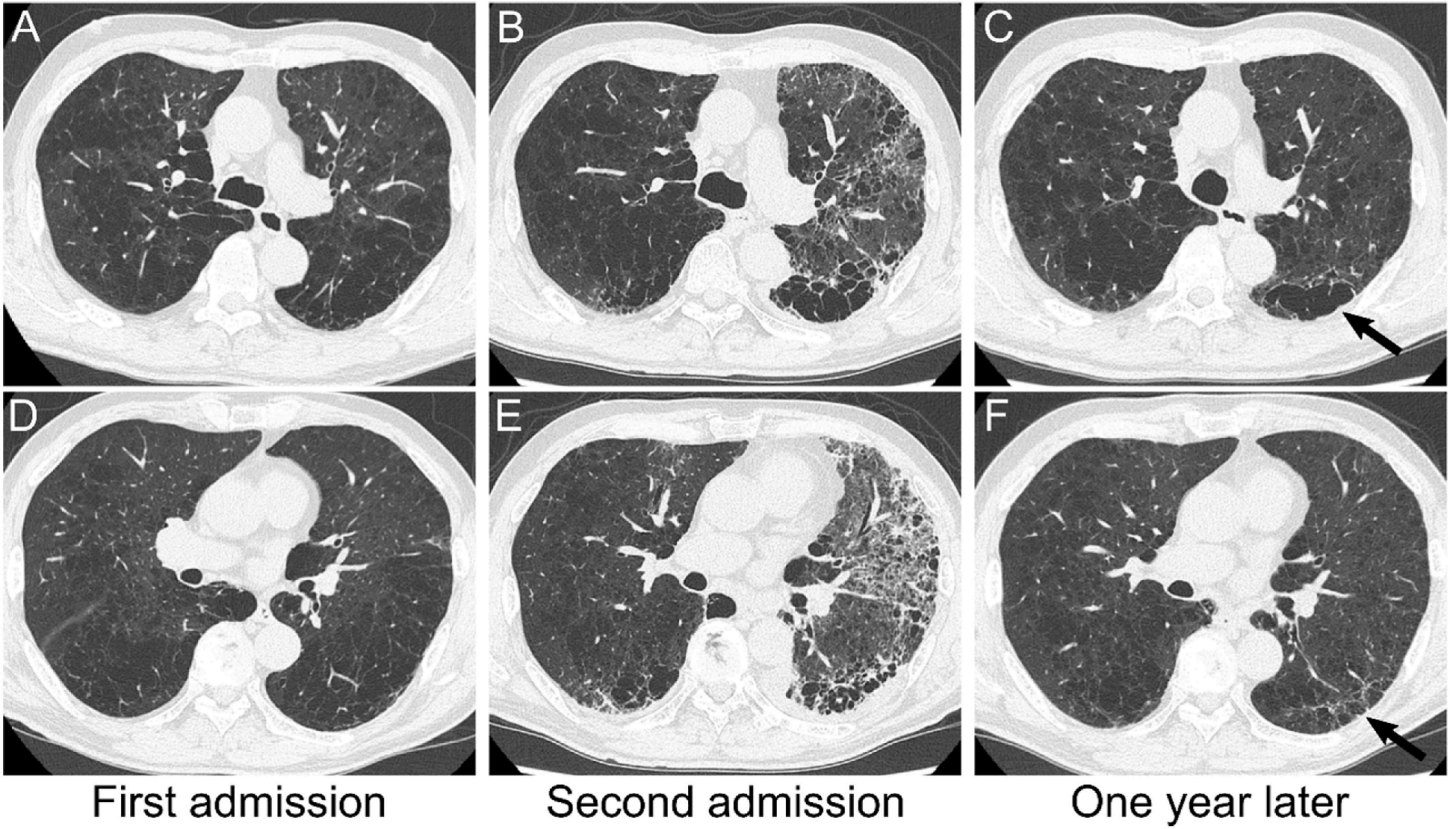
Chest CT findings on the first admission, second admission, and at one year later. (A and D) On the first admission (day 1), chest CT showed a slight increase in lung densities in the lower lobes. (B and E) On the second admission, chest CT showed opacities in the left lung. (C and F) One year after COVID-19, the emphysematous lesions in the left lung significantly shrank (arrow). CT, computed tomography; COVID-19, coronavirus disease 2019.
